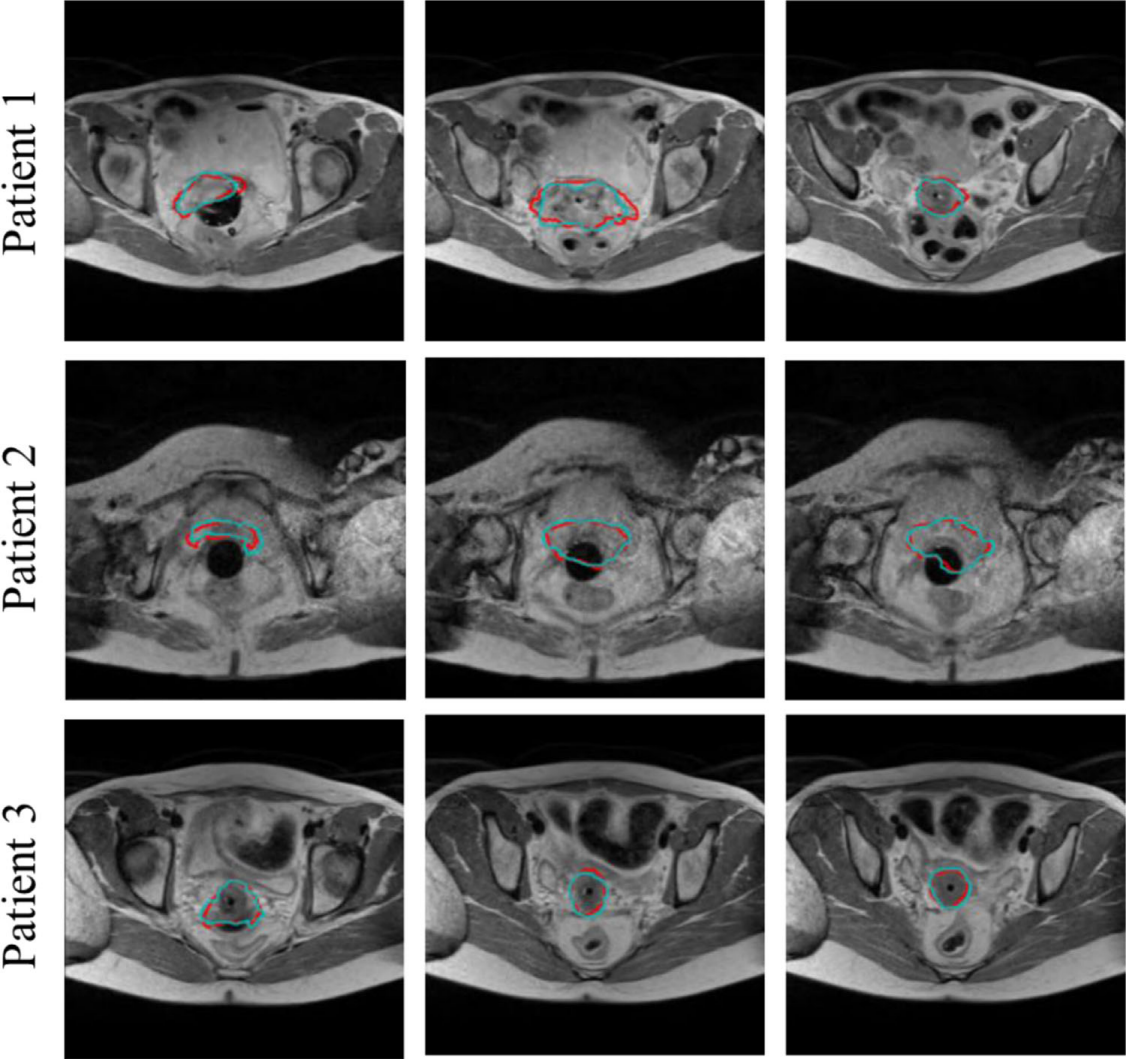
Examples of clinical target volume (CTV) segmentations from MR images using the proposed method: (red) automatic segmentation, (cyan) manual segmentation
